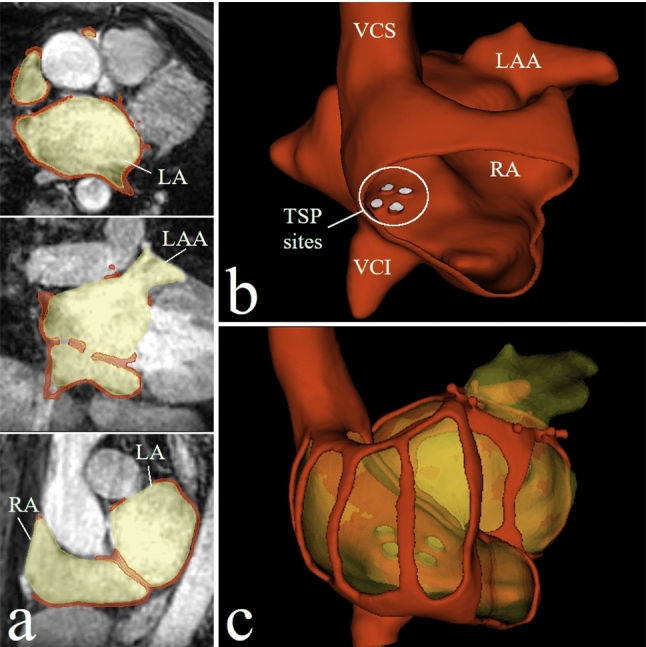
a Segmentation (yellow) of the left atrium (LA), left atrial appendage (LAA), and right atrium (RA) based on the underlaying MRI image volume (transversal, coronal, and sagittal plane) with the resulting base model (red). b TSP sites in the base model with vena cava superior (VCS), vena cava inferior (VCI), RA, and LAA as landmarks, c base model (red) in 3D space with the underlaying segmentation (yellow)
